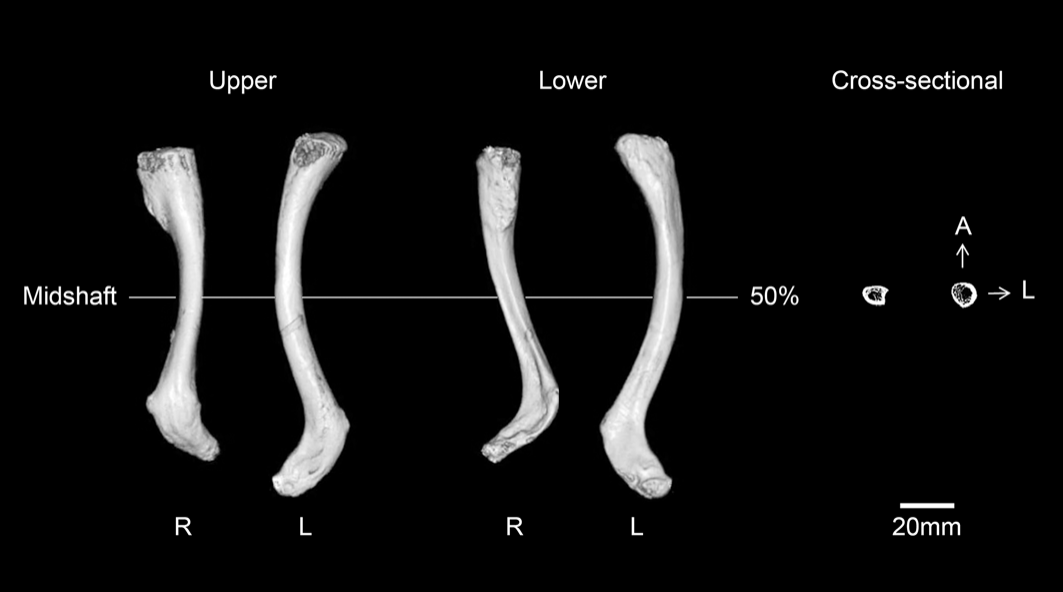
Reconstructed CT images and cross-sectional images of the clavicles (A, anterior; L, lateral).
